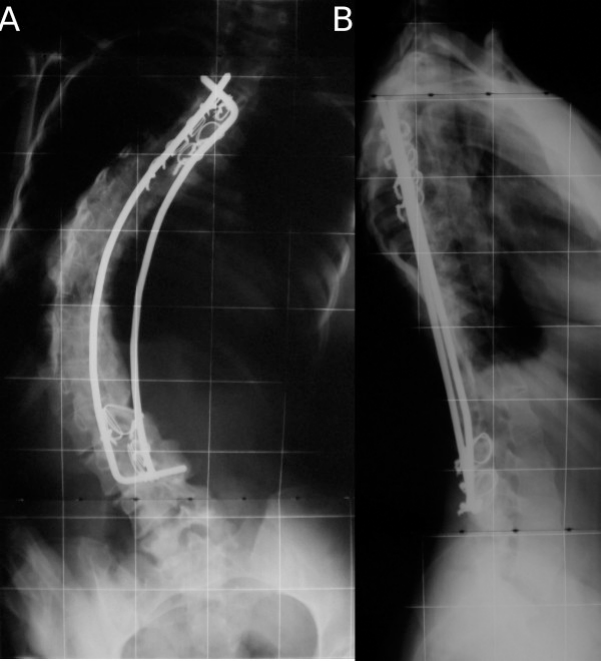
(A, B), Case 2: Spine radiographs at a 6-year follow-up; anteroposterior (A) and laterolateral (B) view.
